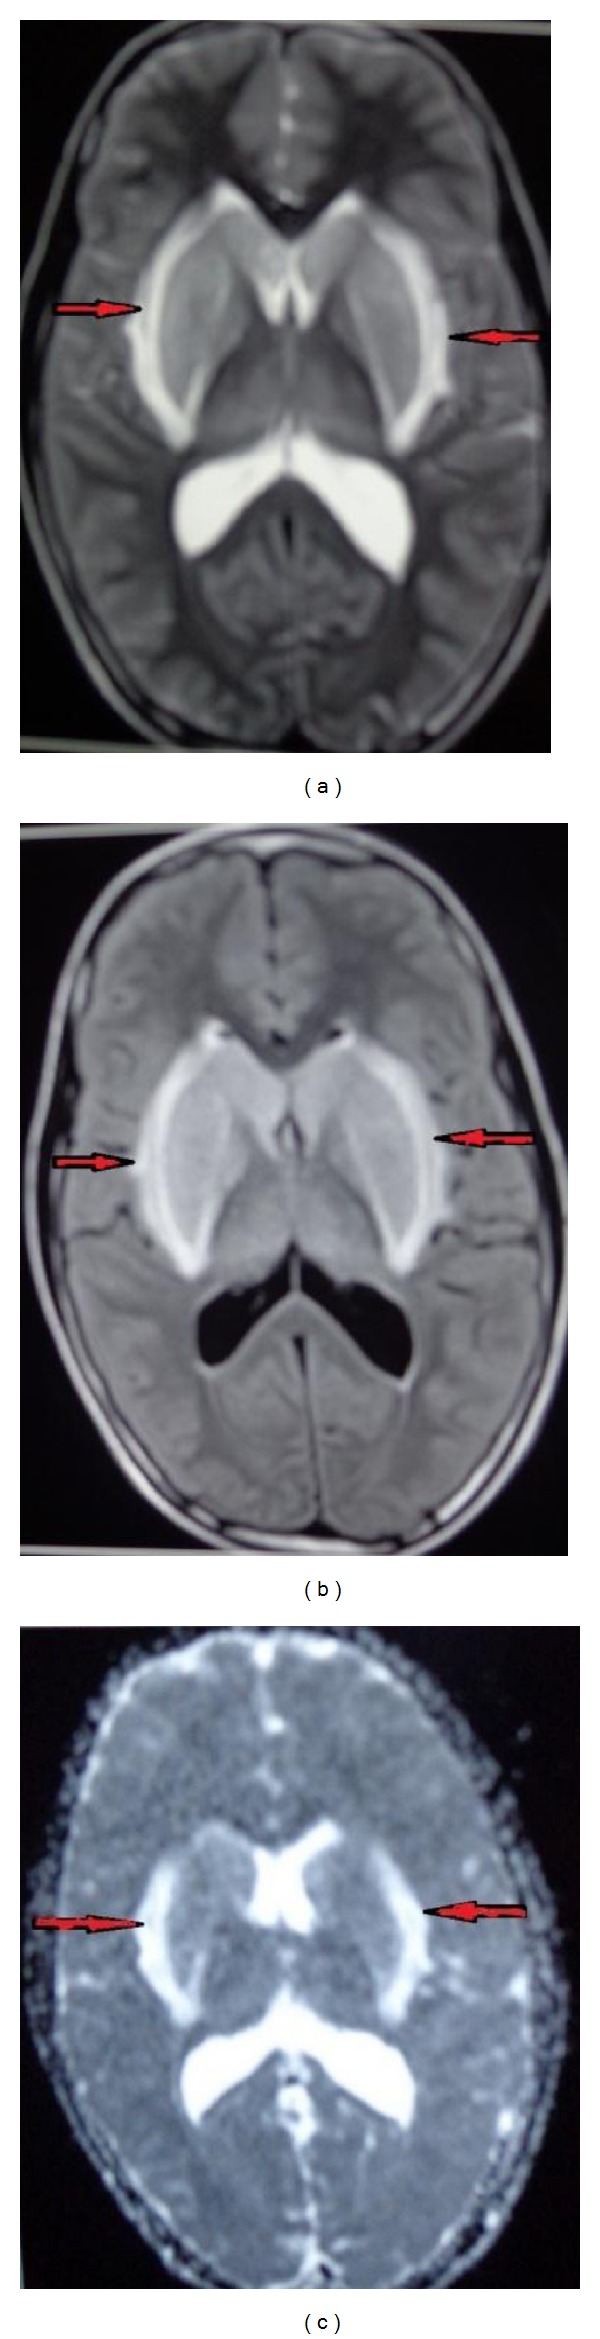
((a), (b), and (c)) Brain MRI (T1, T2, DWI) showed bilateral basal ganglia are grossly swollen with vasogenic edema tracking along internal capsule and midbrain. Adjacent ventrolateral thalamus and internal capsule also show mild abnormal intensities.
